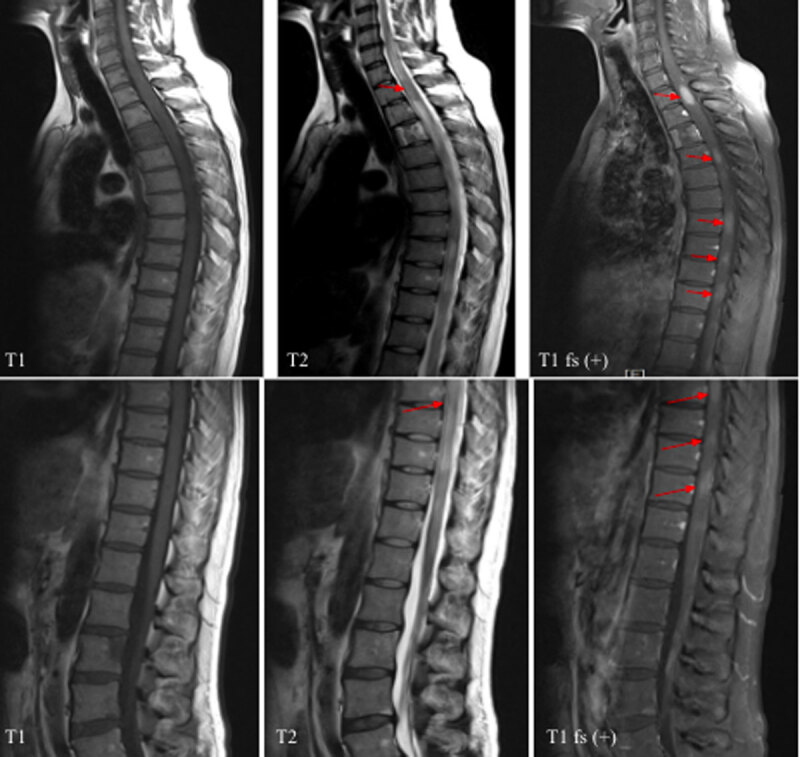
Pre- and post-contrast MRI: Multiple enhancing spinal cord lesions with isointense on T1WI and iso/hyperintense on T2WI (arrows).
